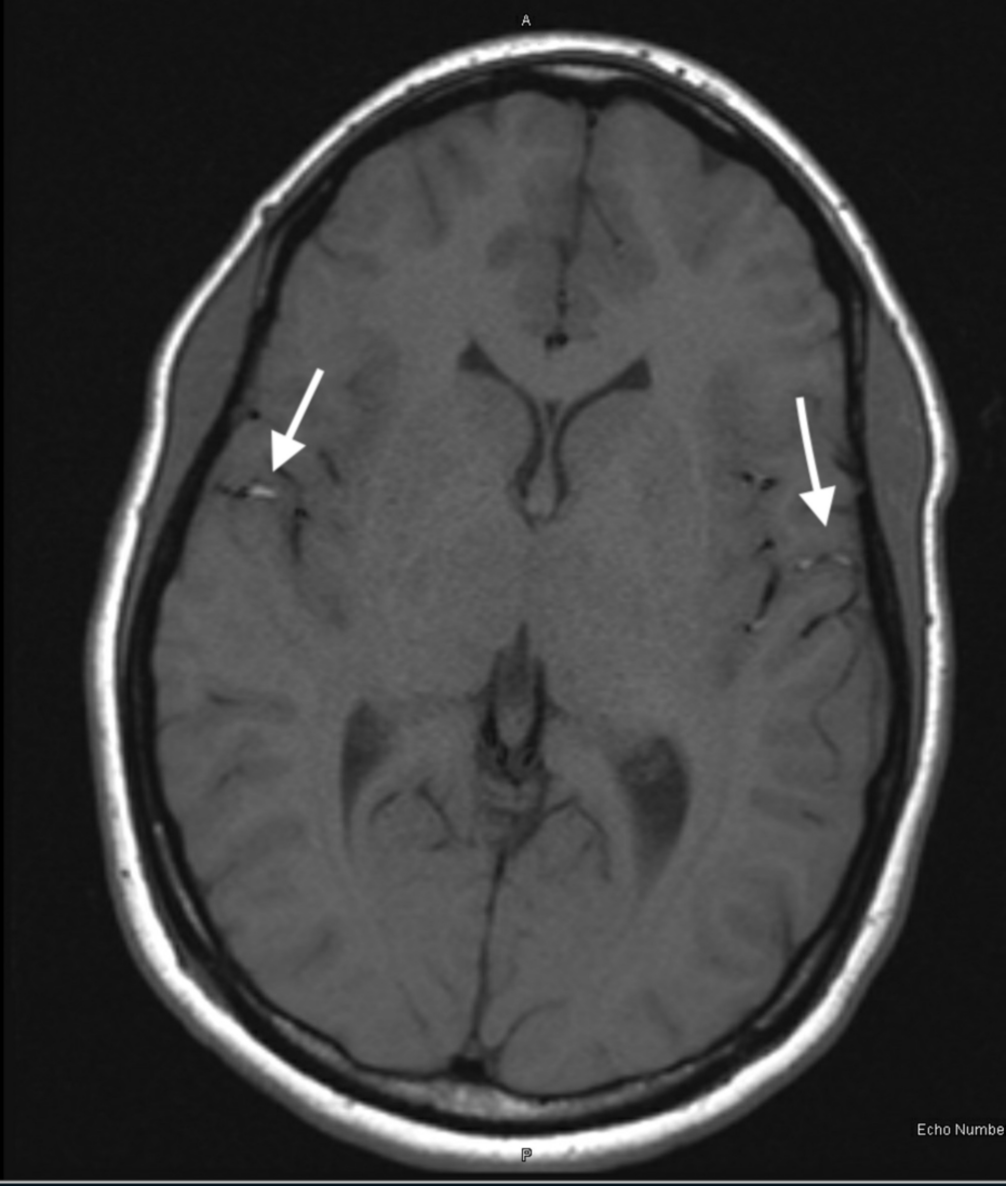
Axial T2 Flair MRI Brain
